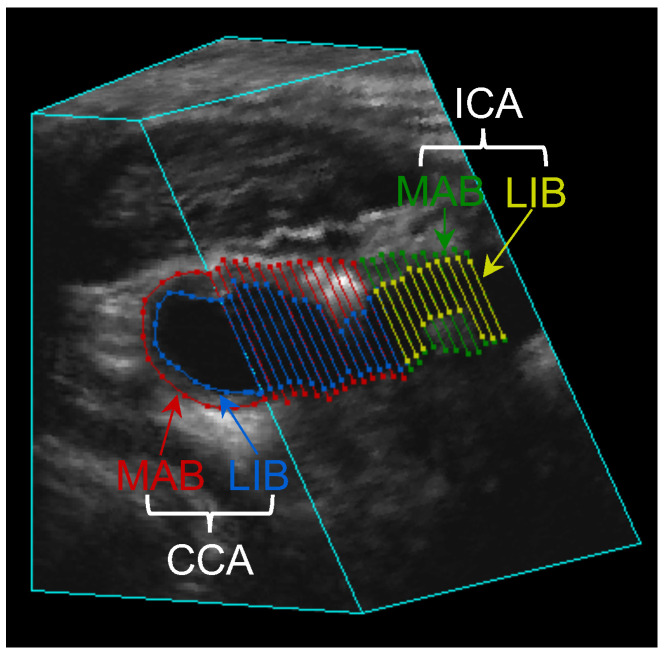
The 3D ultrasound image with MAB and LIB of the CCA and ICA segmented at the 1 mm ISD setting.
